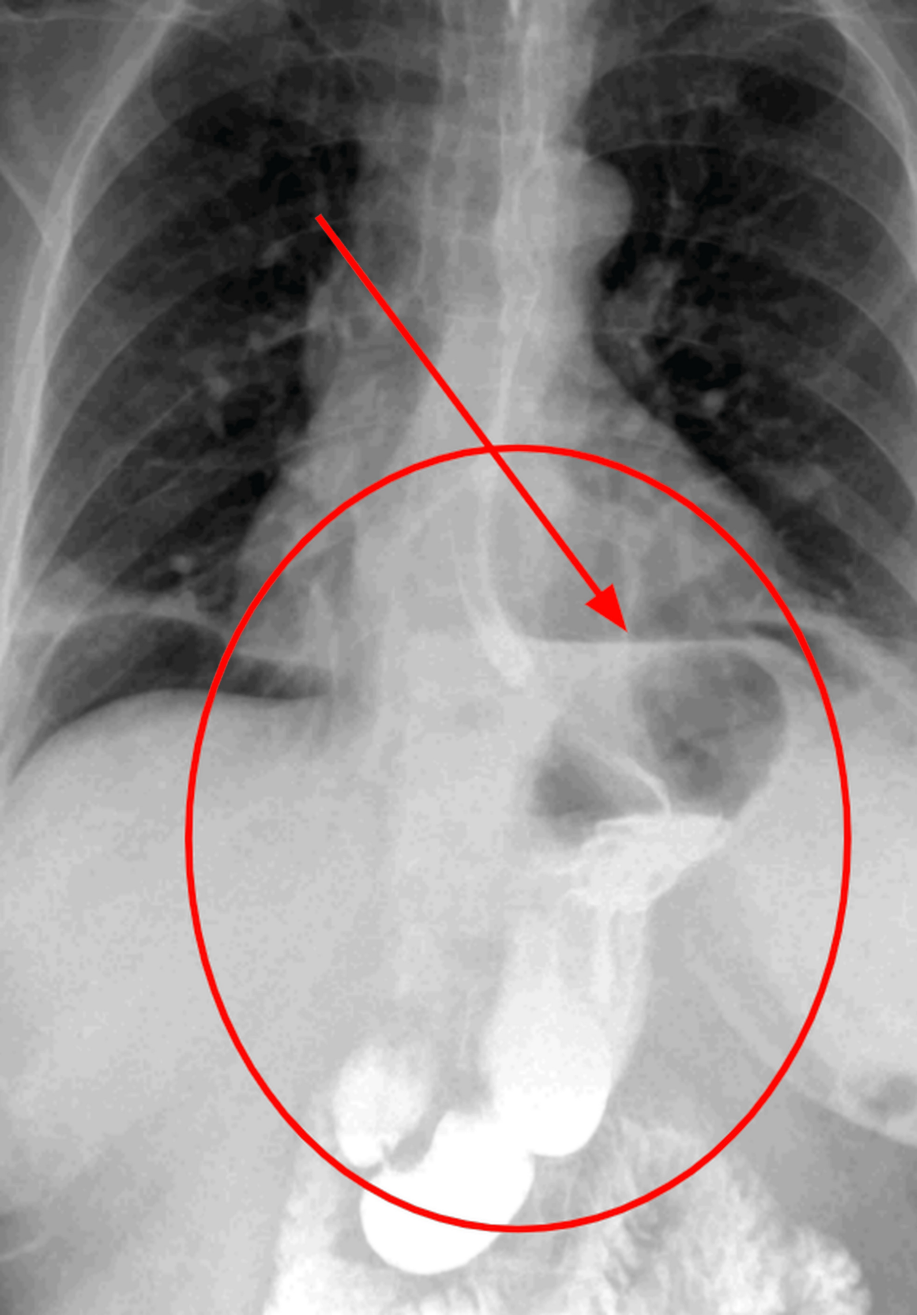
Upper gastrointestinal contrast study showing a large intrathoracic stomach. A frontal radiograph with oral contrast demonstrates a markedly distended stomach herniated into the thoracic cavity (outlined by the red circle), consistent with a giant hiatal hernia. The red arrow indicates the transition point between the esophagus and the volvulized portion of the stomach, suggesting organoaxial gastric volvulus. Air-fluid levels and abnormal gastric positioning above the diaphragm are evident, supporting the diagnosis. This imaging finding correlates with the patient's acute epigastric pain and clinical signs of gastric obstruction.
